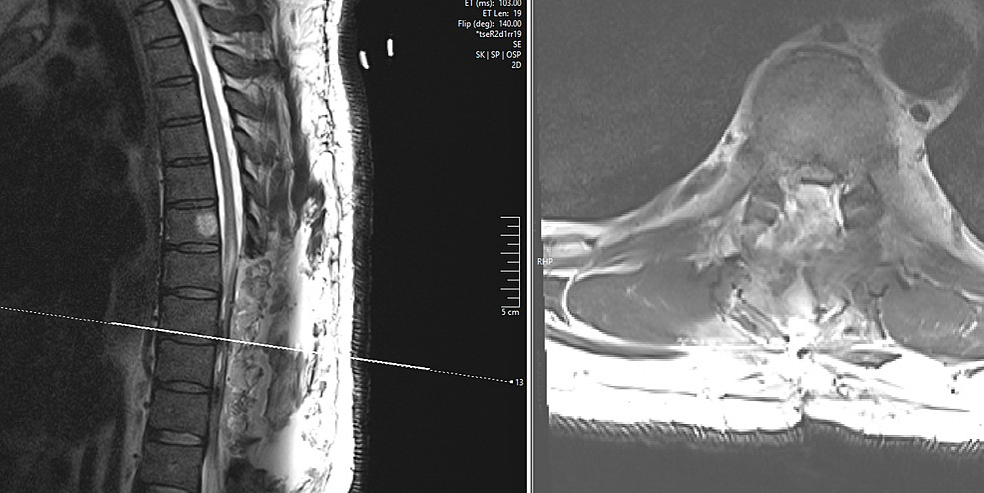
MRI in T2-weighted sequences one week following gross total resection of a T7-9 intramedullary spinal cord ependymoma. Images in sagittal (left) and axial (right) planes demonstrate resection of the intramedullary mass and mixed intensity fluid impressing upon the dura posteriorly, thought to be a mixture of CSF and blood product. Fluid appears to extend into the right lateral T8/9 recess where the dural tear had occurred, though no definitive defect in the dura is visible on MRI. MRI: magnetic resonance imaging; CSF: cerebrospinal fluid
